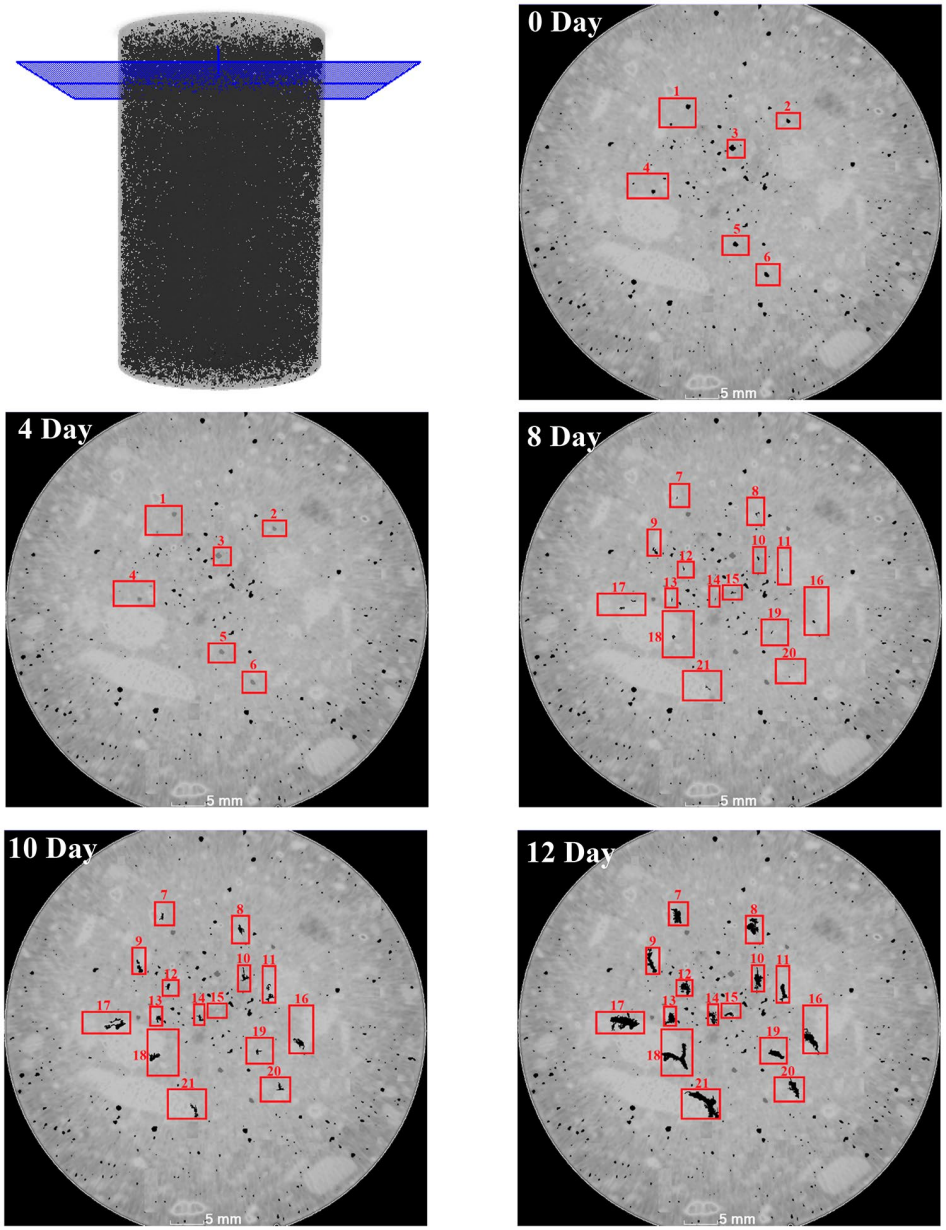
CT images depicting the pore structure in region without the reinforcing bar during various stages of accelerated corrosion.
